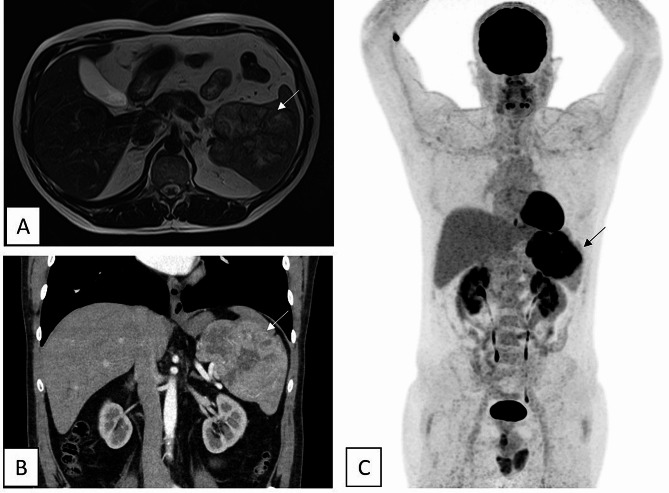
Imaging evaluation after 4 years of the initial symptomatology showing a 106 mm lobulated heterogenic and hypermetabolic splenic mass. A : Axial abdominal MRI. B : Frontal abdominal CT-scan. C : Whole body TEP-scan (SUVmax = 16.9). Arrows indicating splenic tumour mass with no other identified lesion
